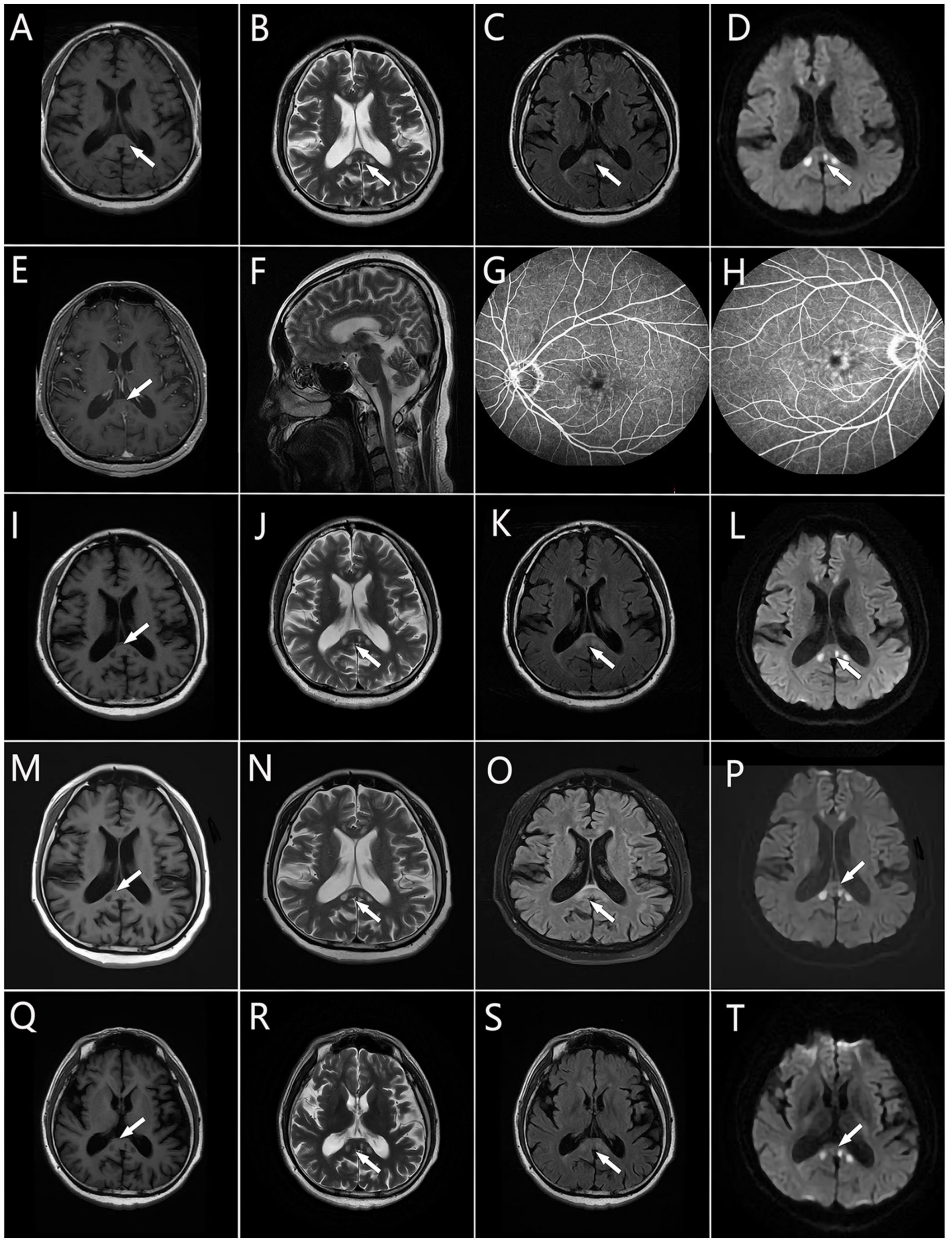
Brain MRI. (A,I,M,Q) Multiple round hypointense lesions on T1 in the corpus callosum. (B,F,J,N,R) Multiple round hyperintense lesions on T2 in the corpus callosum. (C,K,O,S) Multiple round hyperintense lesions on FLAIR located in the corpus callosum. (D,L,P,T) Multiple hyperintense lesions on DWI located in the corpus callosum. (E) Multiple hyperintense lesions on contrast-enhanced MR located in the corpus callosum. (G,H) The macular capillary was dilated and there is fluorescein leaking from the dilated blood vessels. Areas of hyperintensity or hypointensity are denoted by the white arrows.
